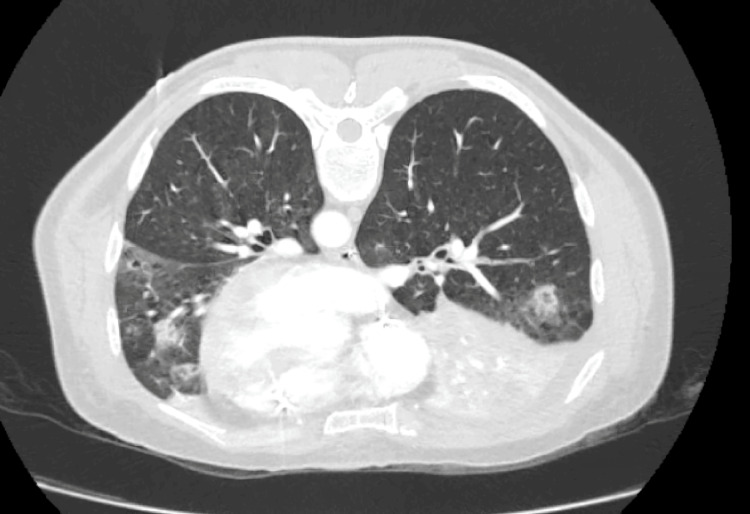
CT pulmonary angiography (axial, lung window) on ICU day 4 Mild posterior ground-glass opacities (boxes) with otherwise preserved aeration is seen. No proximal pulmonary arterial filling defects seen on this slice. Digital markers: boxes highlight ground-glass areas. CT = computed tomography; CTPA = CT pulmonary angiography
